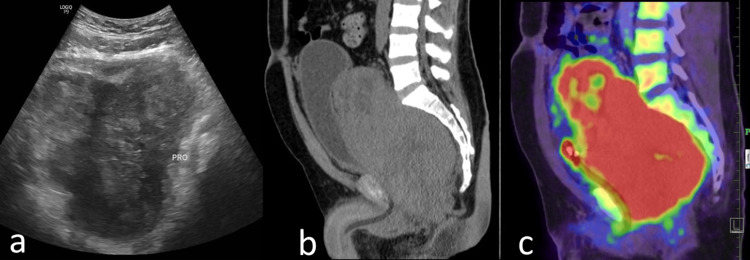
Ultrasound image (a) shows a heterogeneous mass lesion with ill-defined necrotic areas within completely replacing the prostate. The sagittal non-contrast CT (b) shows a large heterogeneous soft tissue mass with central necrotic areas arising from the prostate and infiltrating the urinary bladder anteriorly and rectum posteriorly. This lesion is seen to be intensely FDG-avid on PET-CT scan (c). PET-CT: positron emission tomography-computed tomography, FDG: fluorodeoxyglucose, CT: computed tomography
